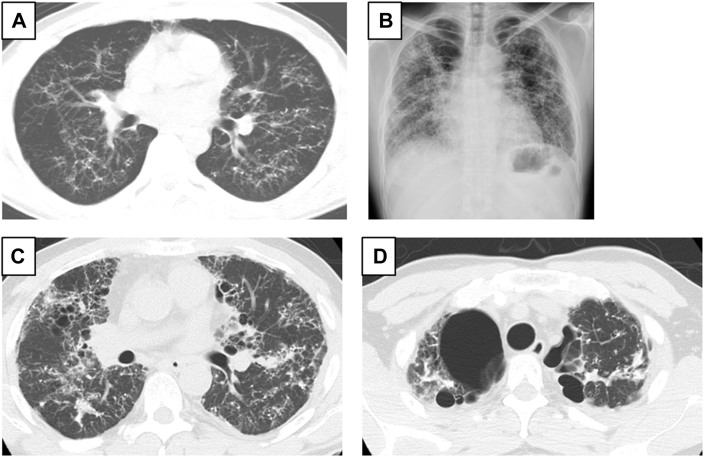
(A) Chest computed tomography images show numerous high-density nodules. (B) Chest radiograph showed worsening infiltration. Chest CT images shows increased micronodules (C) with bullous formation (D).
